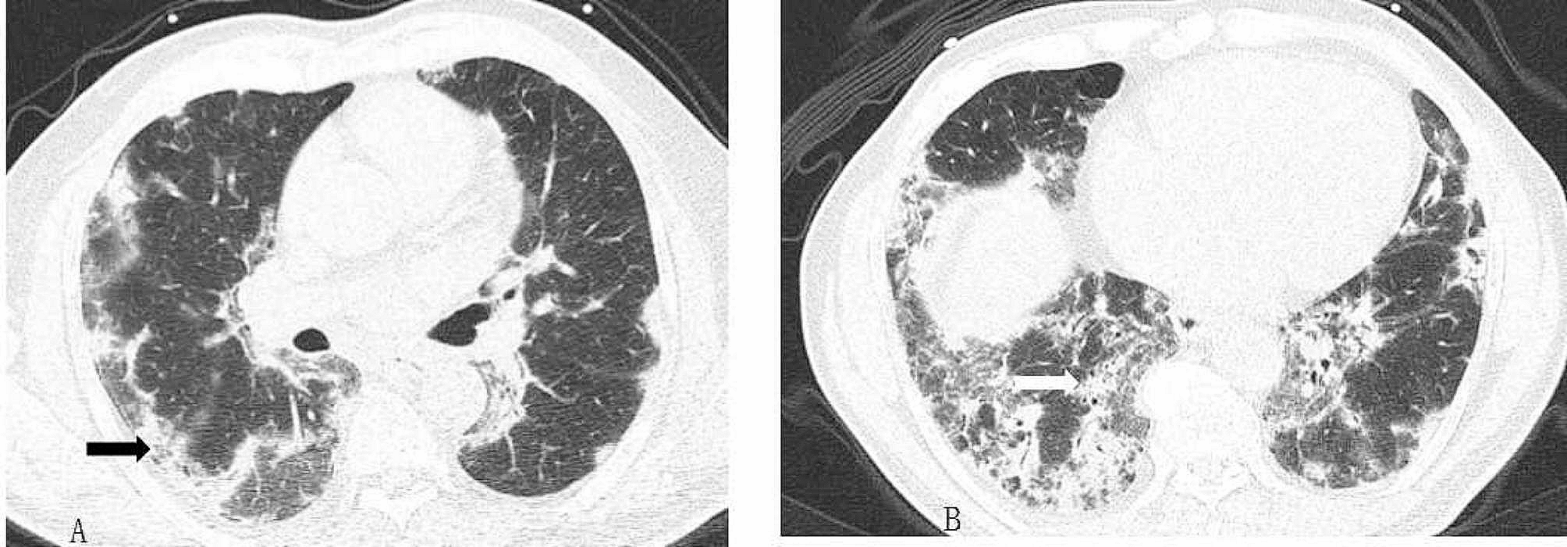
A-B, chest CT scan revealing multiple multifocal, subpleural, bilateral opacities (black arrow, A) and infiltration around the bronchovascular bundle in the bilateral lower lung field (white arrow, B)
